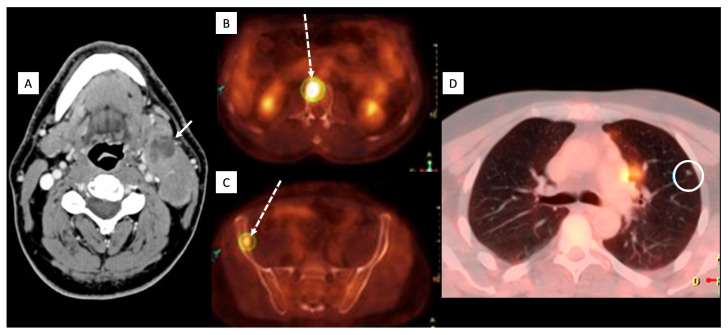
Neck CT and body PET-CT of Patient 2. Axial contrast-enhanced neck CT (A) shows left laterocervical necrotic lymph nodes (arrow). Body PET/CT (B–D) shows increased FDG uptake in lumbar body vertebra, right iliac wing (dashed arrows), and a small pulmonary nodule (circle), consistent with metastases.
